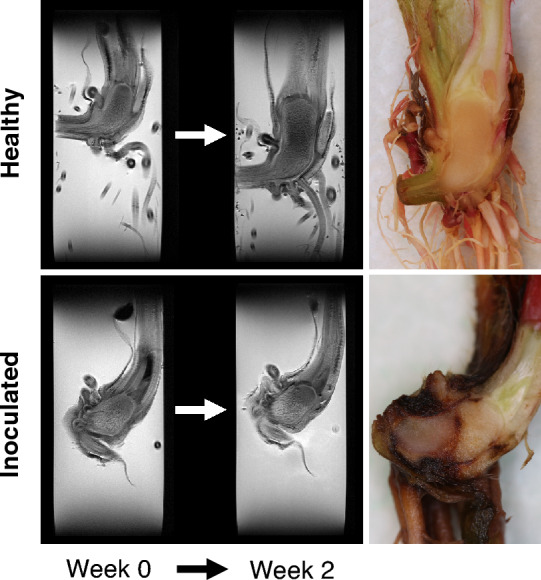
Raw MR images from MEMS data. MR images obtained with multi echo multi slice (MEMS) pulse sequence utilizing the shortest echo time (TE = 9.98 ms) of healthy and inoculated plantlets at different time points. Observe the free water surrounding the plantlets and the limited RF coil range near the upper and lower bounds of the image. The representative examples here are #1 from healthy and #2 from inoculated plantlets presented in Supplementary Fig. S1 online.
